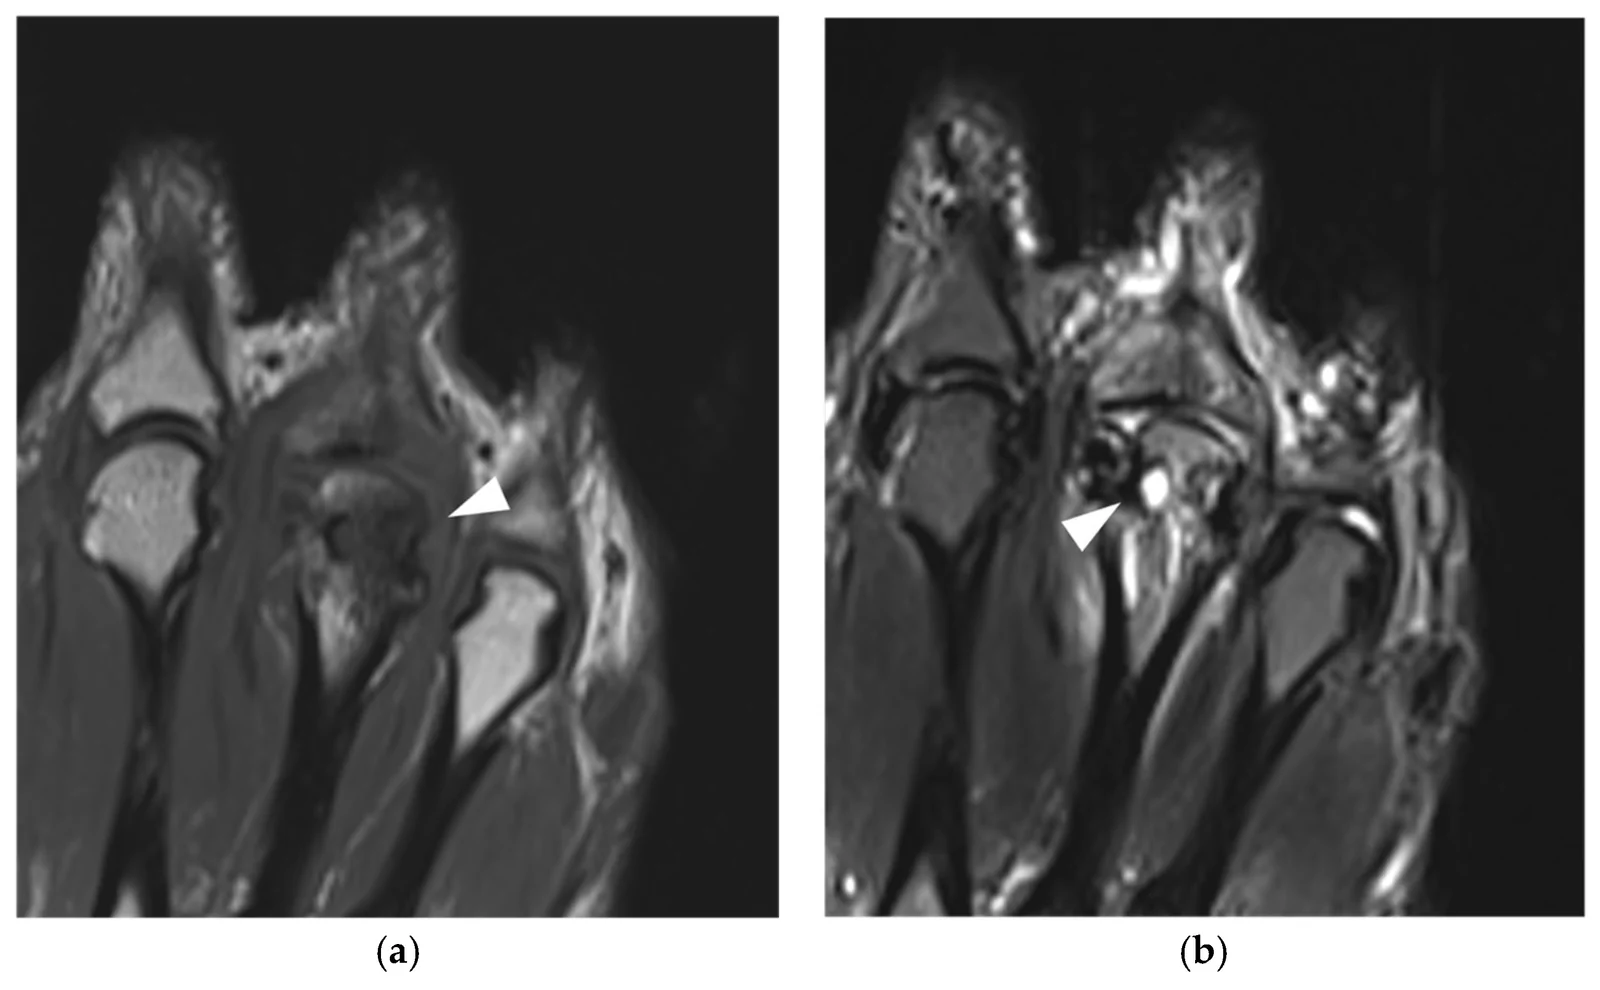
Magnetic resonance imaging (MRI) of the right hand demonstrating subchondral hypointensity on the coronal T1-weighted sequence ((a), arrowhead) and corresponding subchondral hyperintensity on the coronal T2-weighted fat-suppressed sequence ((b), arrowhead) within the third metacarpal head, consistent with avascular necrosis.
